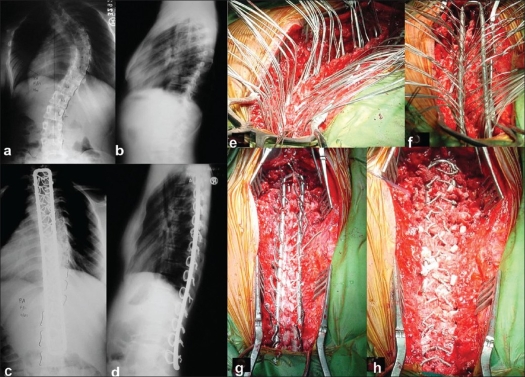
Pre operative (a,b) and post operative (c,d) x-rays of dorso lumbar spine shows good correction, balance and maintenance of a scoliotic deformity at 4 ½ years follow up. Intraoperative photographs show different stages of instrumentation (e-h)
